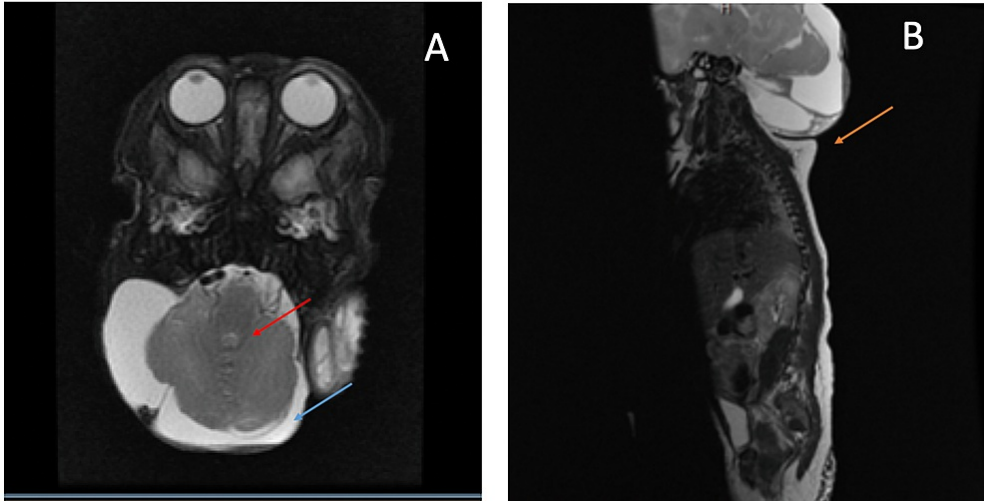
(A) MRI T2 (axial view). (B) MRI T2 (sagittal view). Red arrow shows the encephalocele (hypointense). Blue arrow shows the hydrocele (hyperintense). Orange arrow shows the low occipital/high cervical encephalocele from another angle.
